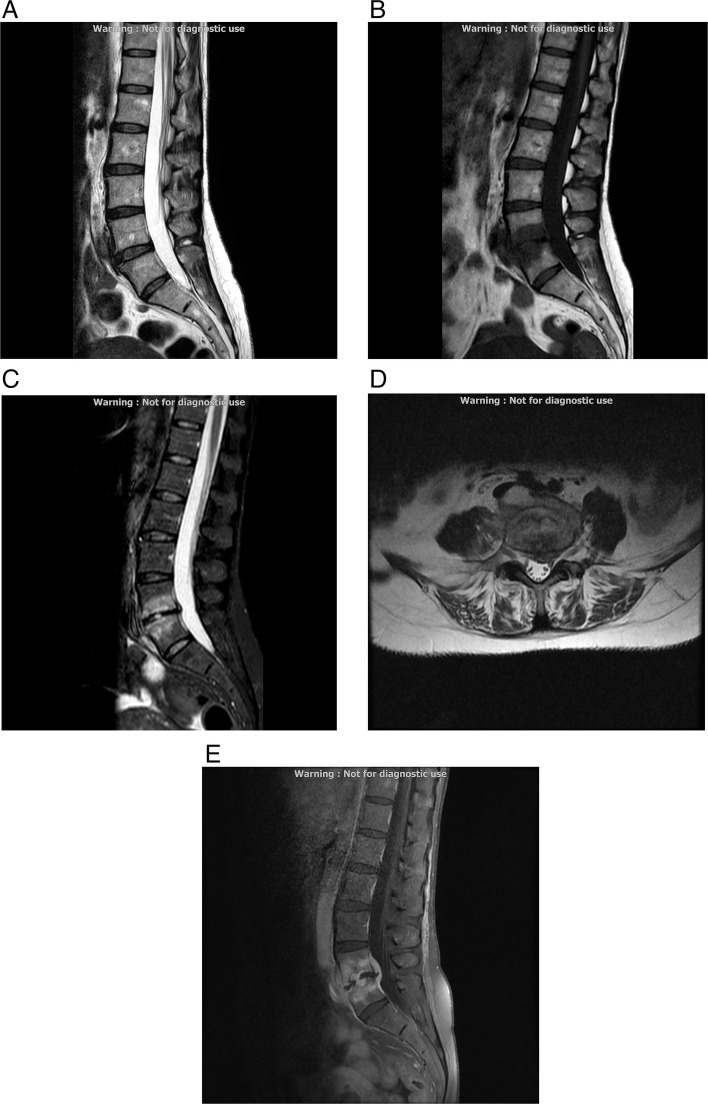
A Sagittal T2-weighted MRI of the lumbar spine demonstrates preserved vertebral body heights and intervertebral disc spaces, without endplate marrow edema or paravertebral/epidural fluid collection. B, C Sagittal lumbar spine MRI demonstrating interval signal abnormality at L5–S1. B Sagittal T1-weighted image shows low T1 marrow signal involving the adjacent vertebral endplates, with mild disc space narrowing/irregular endplate margins. C Sagittal T2-weighted fat-suppressed image demonstrates hyperintense marrow edema and increased disc signal, without significant epidural fluid collection consistent with spondylodiscitis. D, E Follow-up lumbar spine MRI (11 weeks post-procedure) demonstrating progression of L5–S1 spondylodiscitis with extradural collection. D Axial T2-weighted image shows abnormal high signal involving the anterior paravertebral soft tissues, without marked central canal compromise. E Sagittal T1-weighted image demonstrates destructive change centered at L5–S1 with a focal low-signal cavity within the L5 vertebral body and surrounding abnormal marrow/adjacent soft-tissue inflammatory change, compatible with abscess formation
